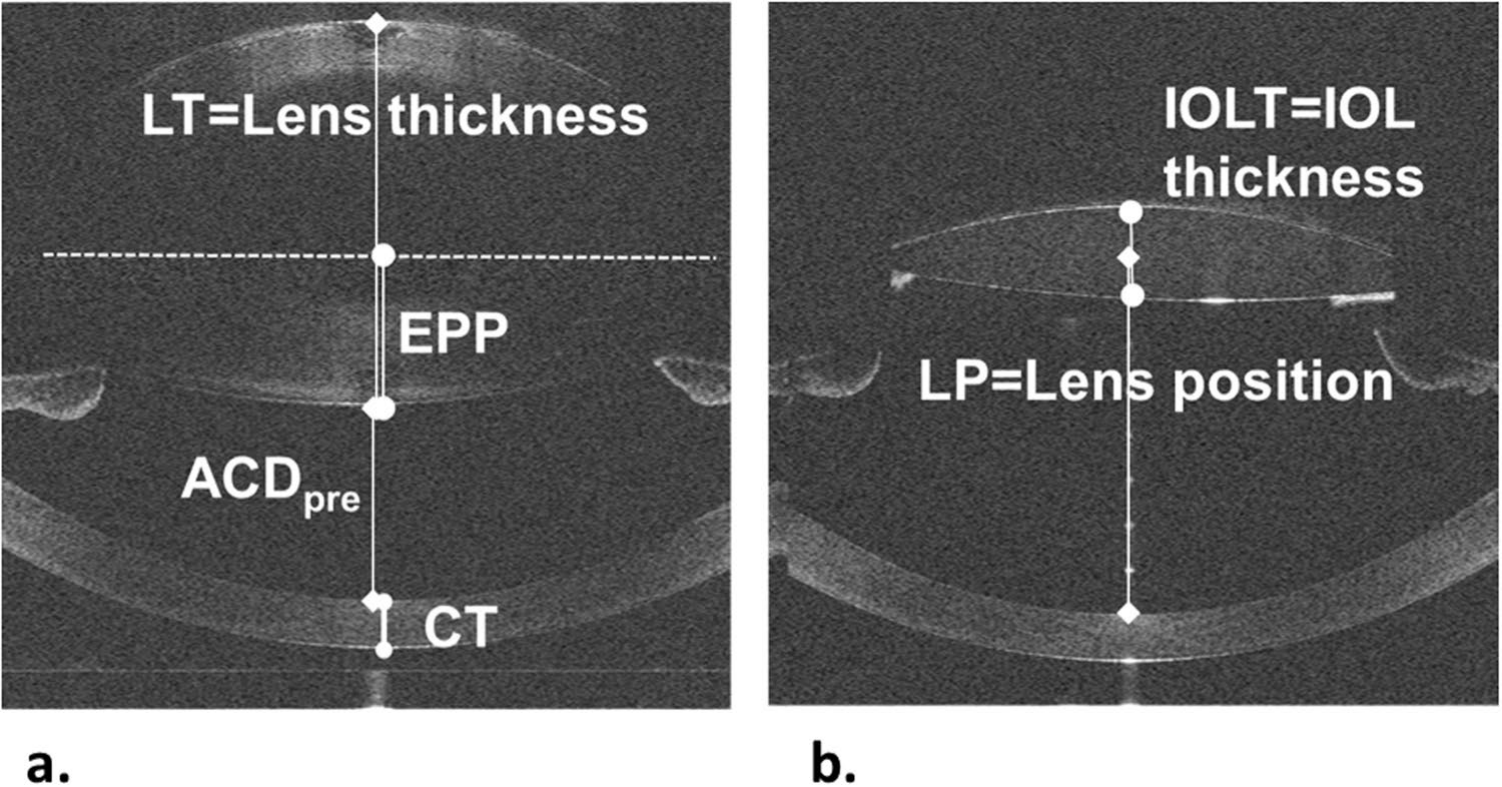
Raw optical coherence tomography (OCT) images for subject S#1 OD, including the definition of some biometric parameters. (a) Preoperative measurement. (b) Postoperative measurement. ACD_pre_ = anterior chamber depth, EPP = equatorial plane position, CT = corneal thickness.
